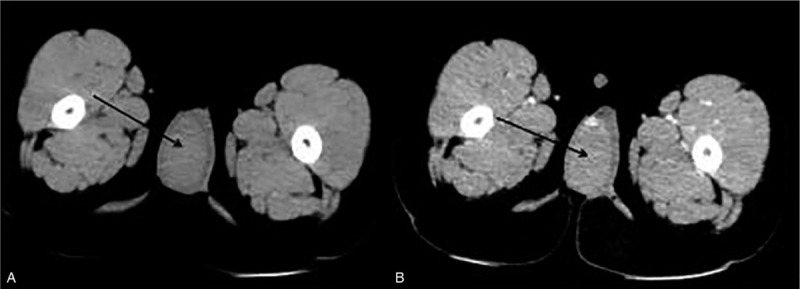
Mass in the right testis by enhanced abdomen CT scan (A: CT scan, B: CT enhanced scan). CT = computed tomography.
